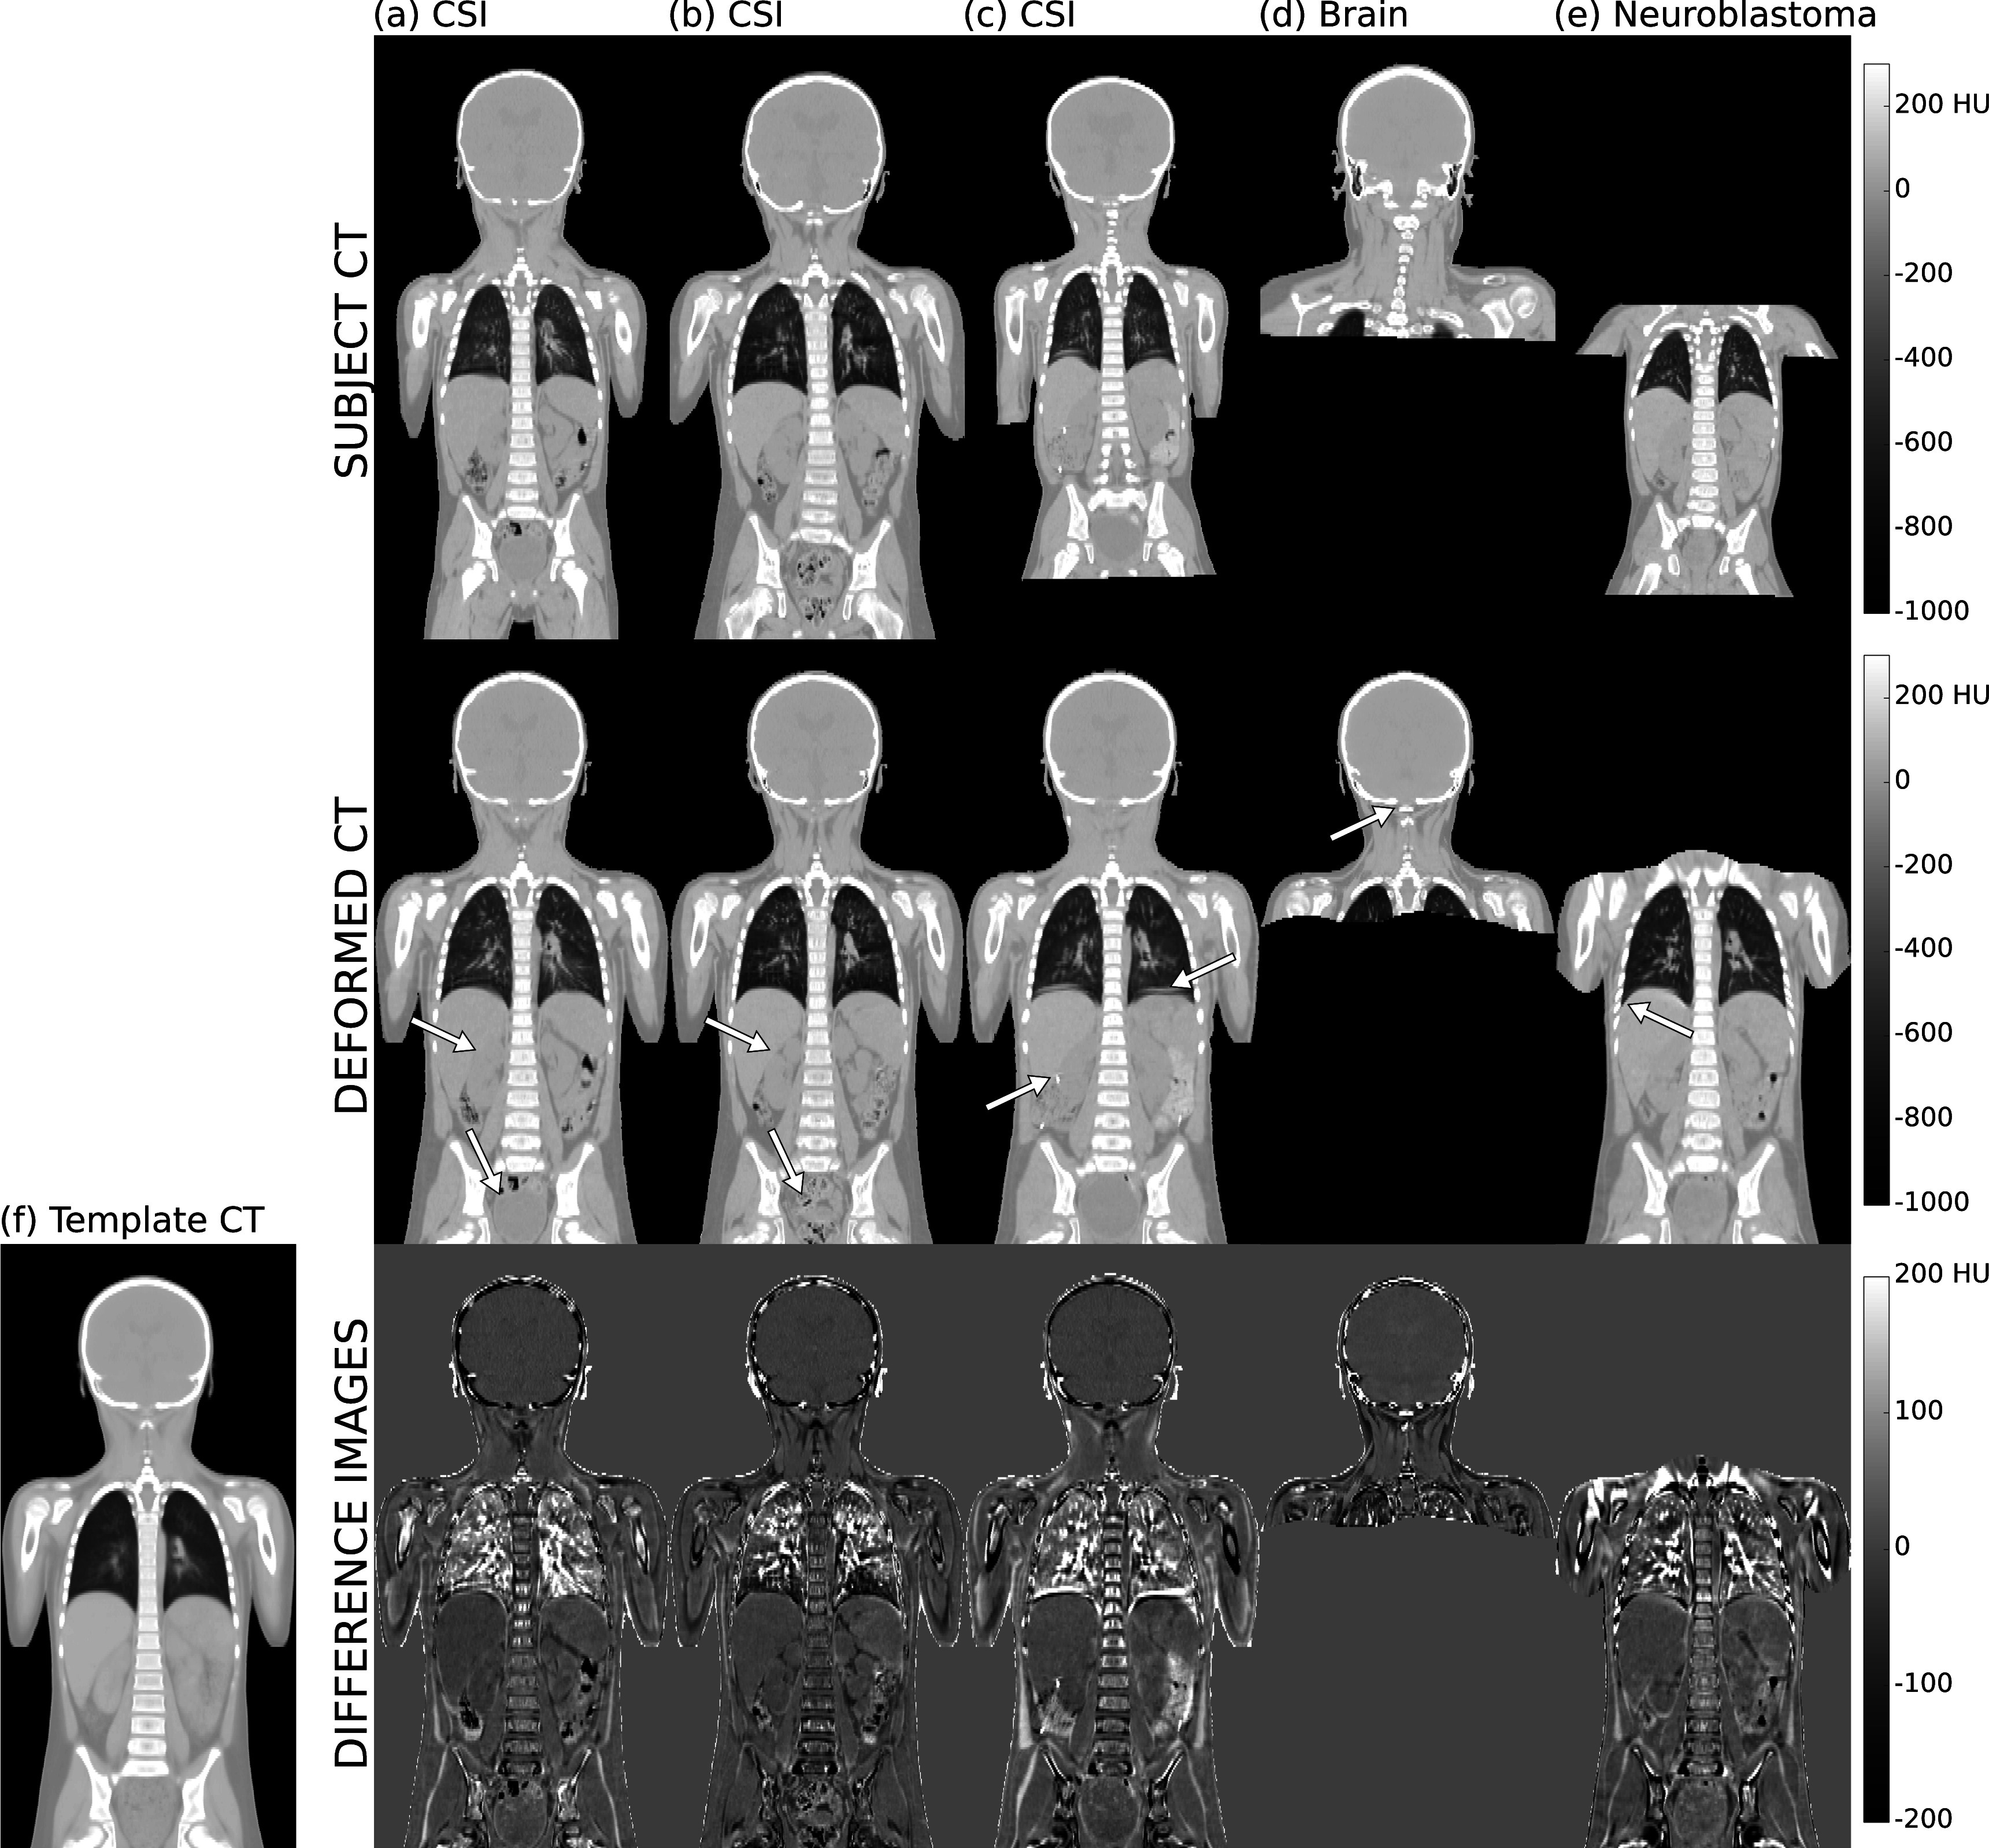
Examples of registrations to the (f) template CT, including subjects from (a)–(c) craniospinal irradiation (CSI) and (d)–(e) other disease cohorts. Top row: subject CT scans (rigid-only alignment). Middle row: deformed CT scans. Bottom row: difference map between registered scans (deformable registration) and template CT. In general, the registrations can successfully align the anatomy, but specific challenges arise in different body regions. Contrast in anatomical boundaries is fundamental to guide the registration, but clinical scan quality may vary. The boundary between liver and kidneys is less sharp in (a) than in (b), for example (top arrows), leading to poorer anatomical matching in this region; likewise, large deformations of the bladder are challenging to recover, where filling varies from (a) full to (b) empty (bottom arrows). Imaging artefacts can also be problematic, such as (c) motion artefacts (top arrow) and/or the use of contrast agents (bottom arrow). Note that high-intensity artefacts were masked out during the registration. Different disease cohorts have differences in image acquisition of parameters, patient positioning and imaged field-of-view ((d) brain tumour versus (e) abdominal neuroblastoma). Arrows indicate examples of misregistration regions on the skeleton (d) and soft tissues (e).
